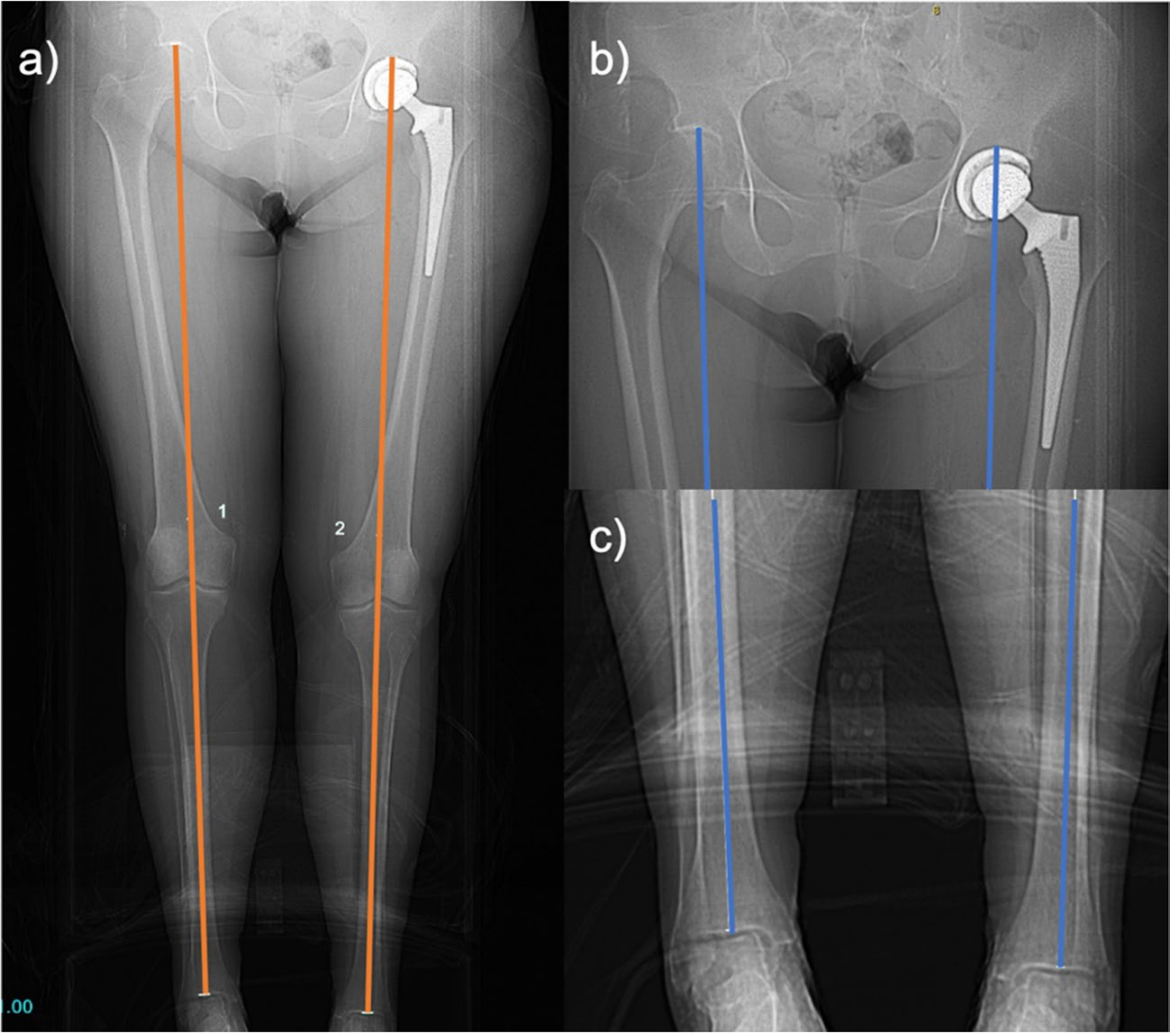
CT scanogram image demonstrating measurement of acetabular apex to tibial plafond distance (AA-TP). a The AA-TP (orange line) was defined as the distance between b the most proximal aspect of either the acetabular prosthesis or sourcil and c the centre of the tibial plafond
